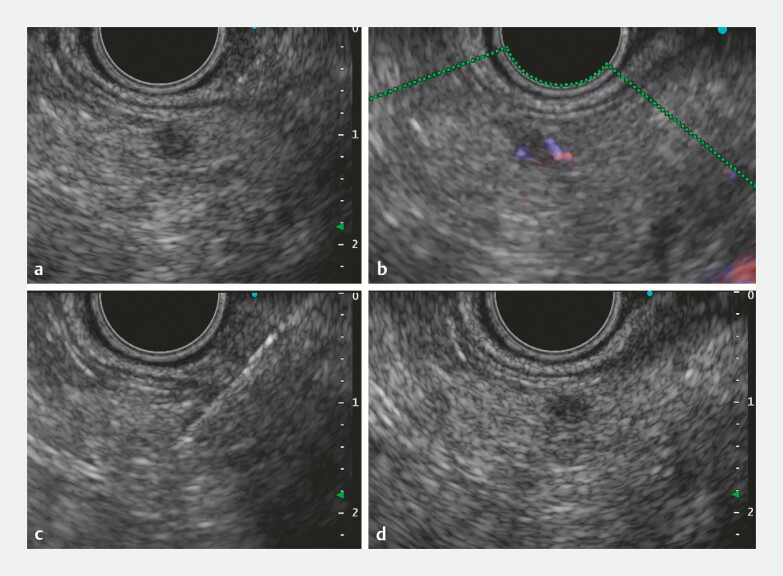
Endoscopic ultrasound-guided fine-needle aspiration of 4-mm pancreatic tumor.
